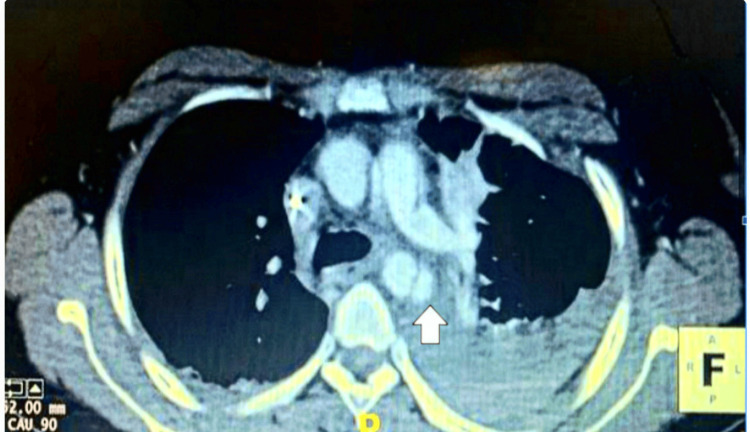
Computed tomography of axial section of the thorax (arrow) shows the presence of aortic dissection of the descending aorta.
